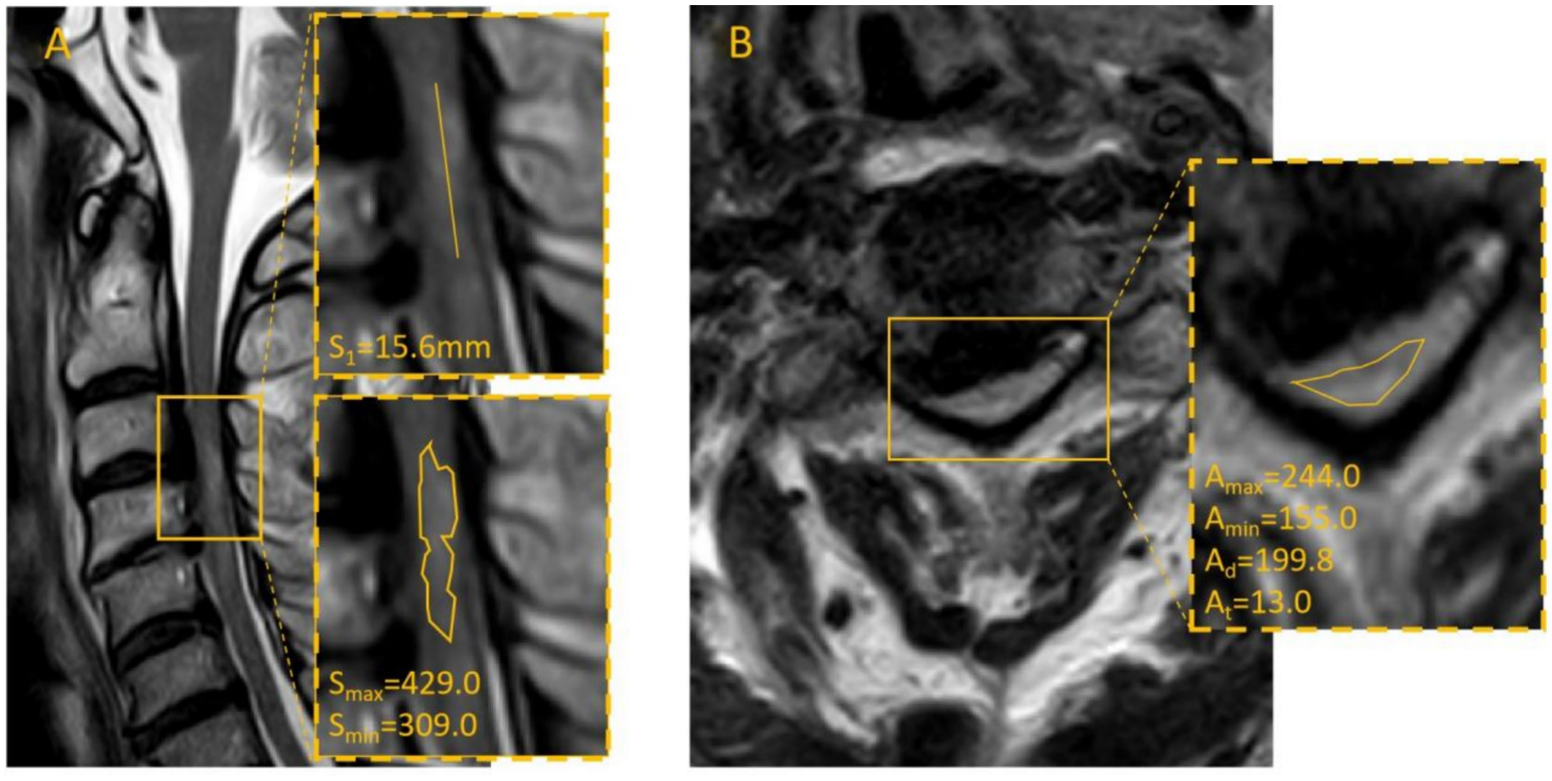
Patient Kang, male, 50years old, admitted 4days after a fall injury. The patient had muscle strength of the four limbs at grade 0–1 with sensoryimpairment; the admission JOA score was 6. The admission cervical MRI is shown in A and B; the measured axial–sagittal difference ratio was 74.17%. The patient underwent five-level posterior cervical surgery. At 3 months postoperatively, the JOA score was 12 and the RR was 54.5%; at 2 years postoperatively, the JOA score was 16 and the RR was 90.9%.
